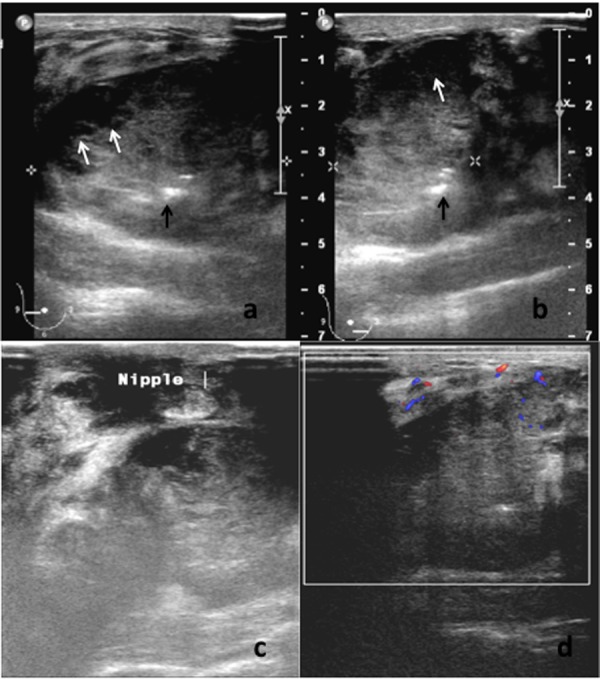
A and B, Gray-scale ultrasound examination of the right breast showing large partially delineated hypoechoic mass containing small cystic areas (white arrows) and echogenic foci (black arrows). The surrounding breast parenchyma showed heterogeneous echotexture; C, The mass is closely related to the nipple causing nipple retraction and fixation; D, Color-Doppler ultrasound study showed low intra-tumoral vascularity with moderate flow signal surrounding the mass.
